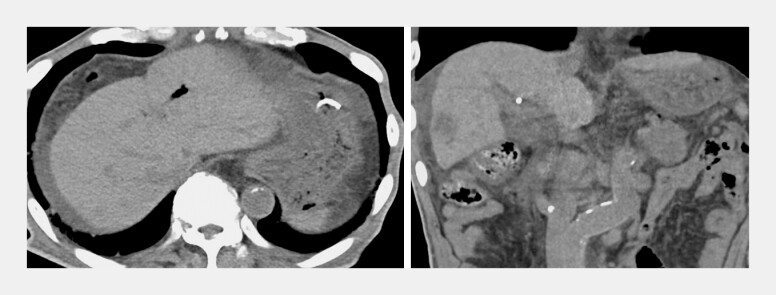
A follow-up abdominal computed tomography scan performed the day after readmission showed the spontaneous resolution of gas within the gastric wall and portal vein.
